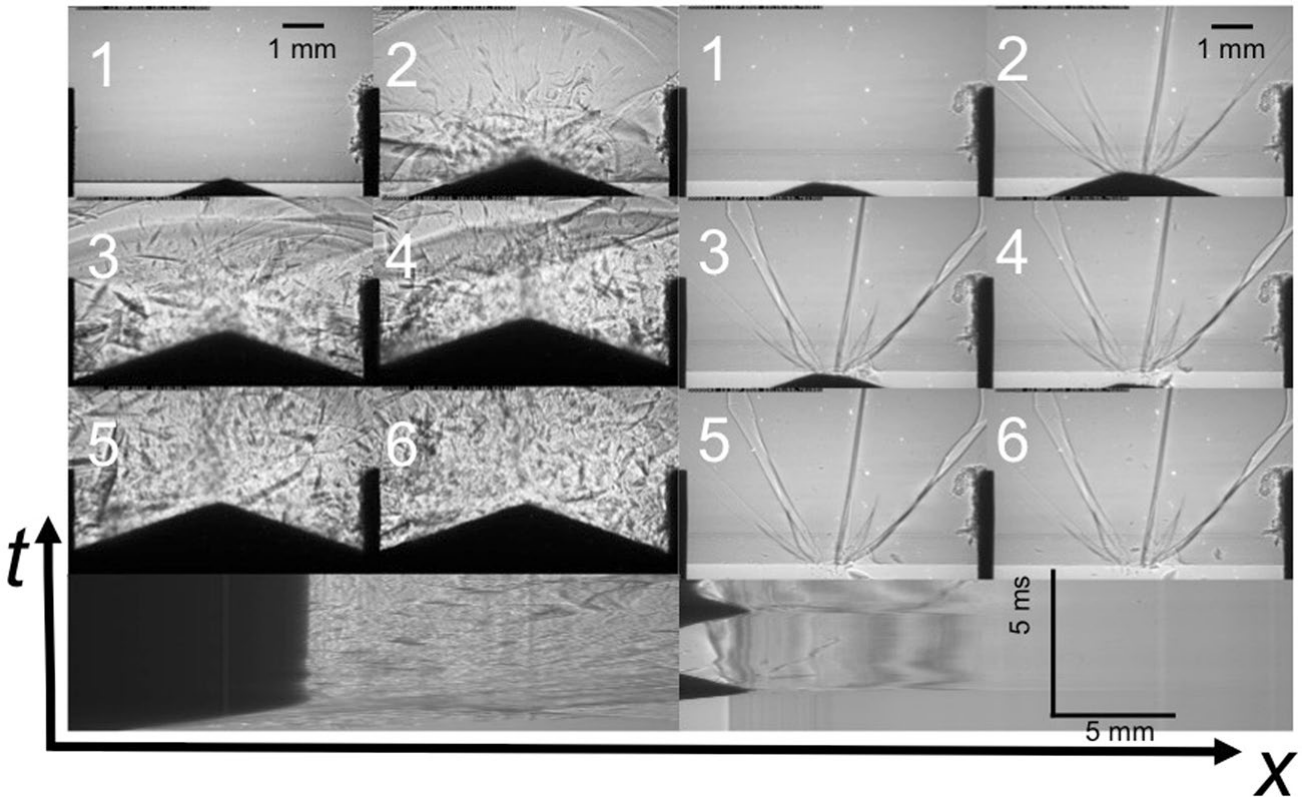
Impact of a triangular prismatic indenter. BS sequence to right and quartz to left. Conditions as for those of Fig. 2. The upper part of the figure shows frames from radiographic sequences whilst below each is a streak image for each impact with dark regions showing the indenter and grey-scale reflecting the density of damaged regions down the central axis of the target. Interframe time *ca*. 68 µs.
